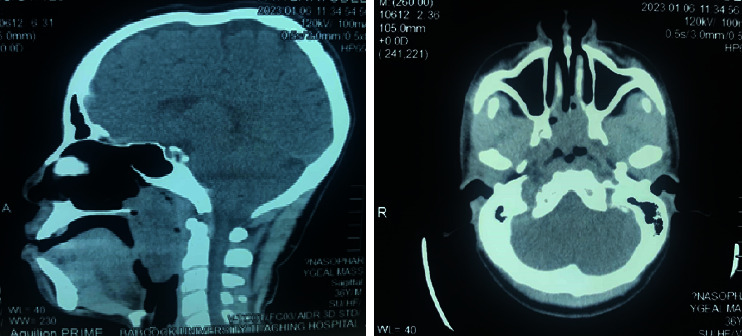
Computed tomography scan images showing a nonenhancing isodense mass lesion occupying the posterior nasal cavity, nasopharynx, and inferiorly to the oropharynx.
